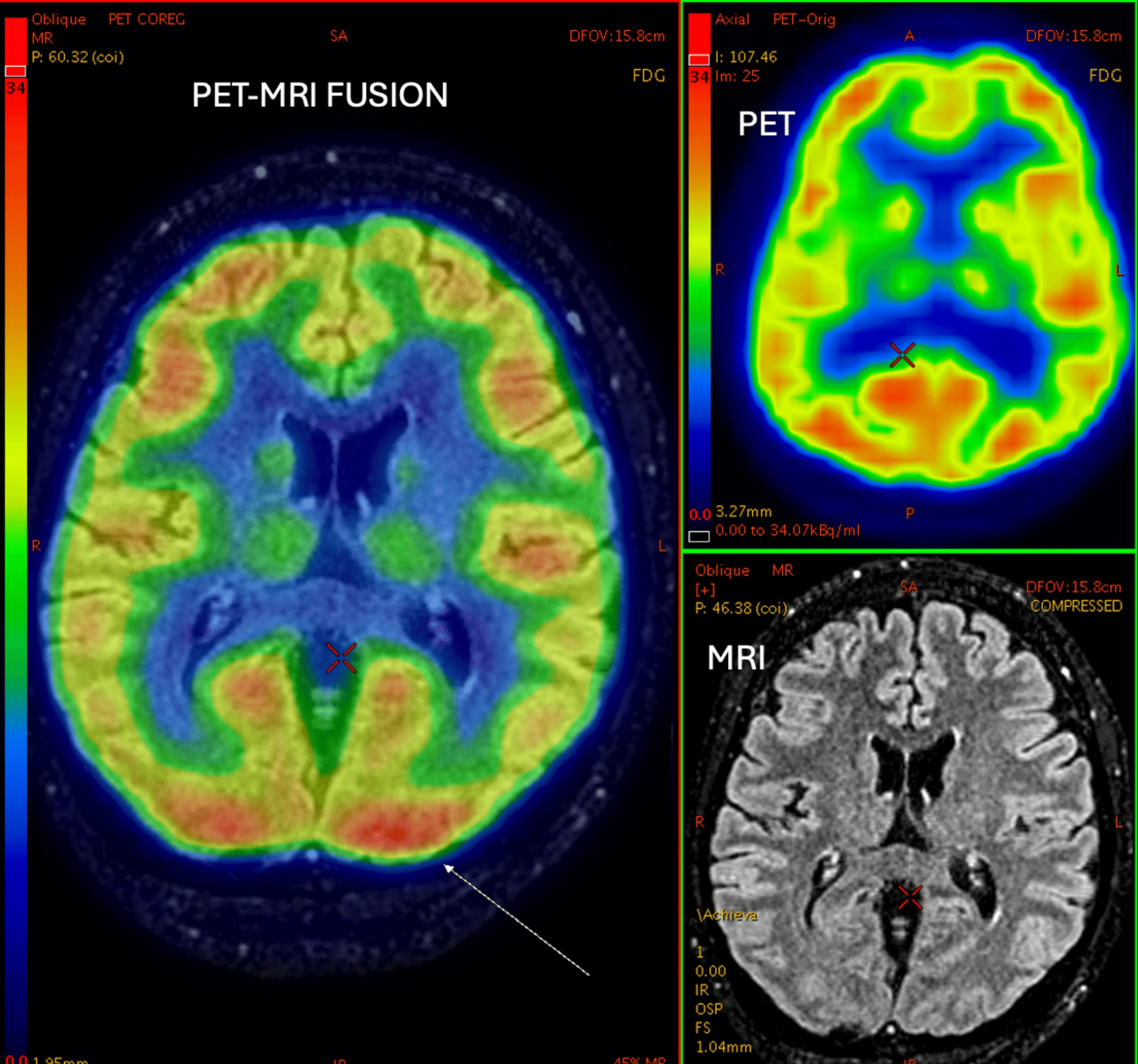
58-year-old male, K/c/o migraine. C/o imbalance, raised morning BP, mild morning headache, and fatigue for 10 days. PET-MRI brain study shows the primary visual cortex on the left side with increased metabolic activity (>2.3 SD). No underlying structural abnormality is seen. PET: positron emission tomography; MRI: magnetic resonance imaging; BP: blood pressure
